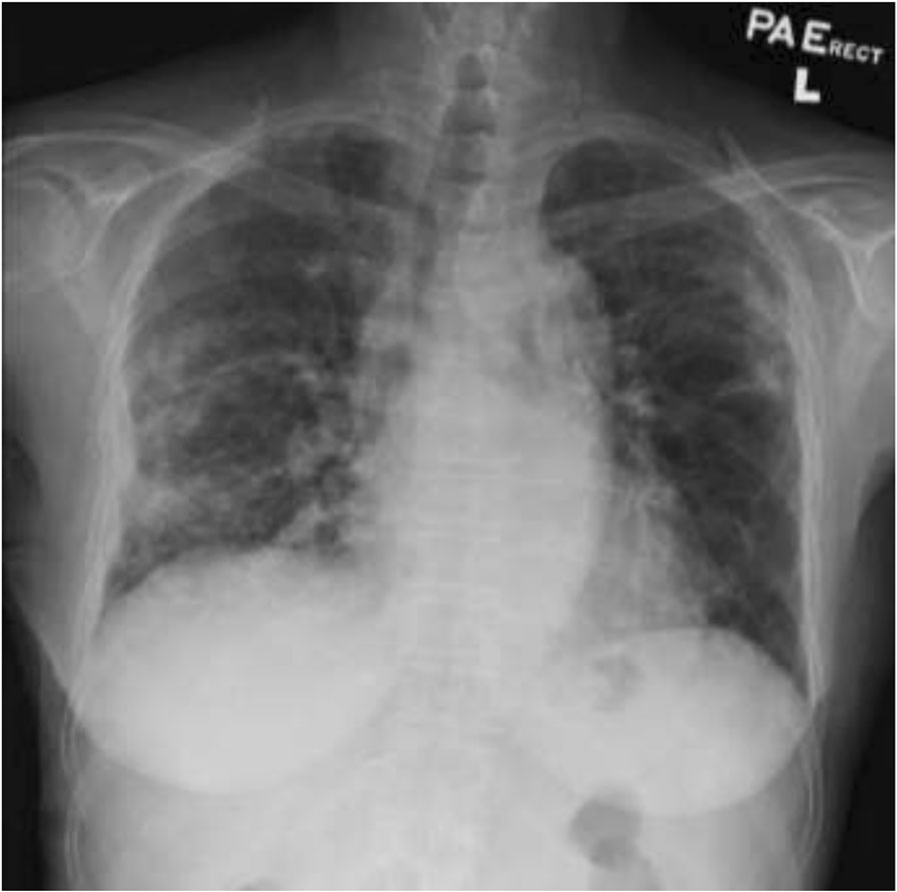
Chest X-ray on discharge showing resolution of the pneumothorax
